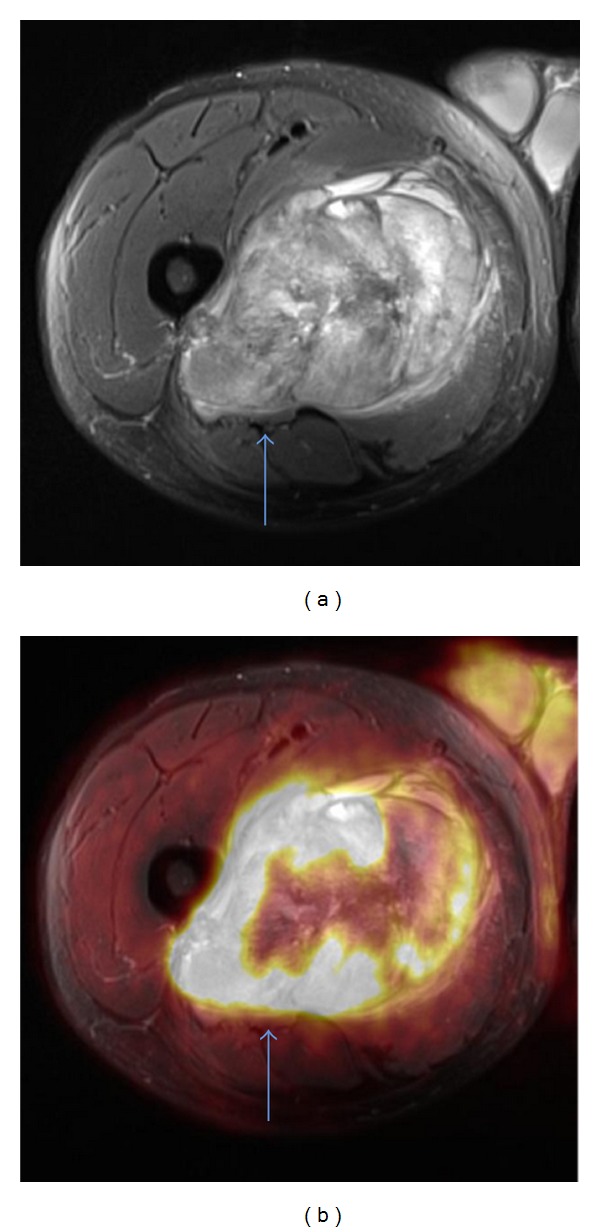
T1-weighted postgadolinium MRI shows a high-grade pleomorphic liposarcoma in the adductor muscles of the right femur (a) and tumor invasion into the sciatic nerve could not be elucidated (Case 2). Fused FDG-PET and MRI show no tumor involvement of the sciatic nerve (b). Sciatic nerve is marked with a blue arrow.
